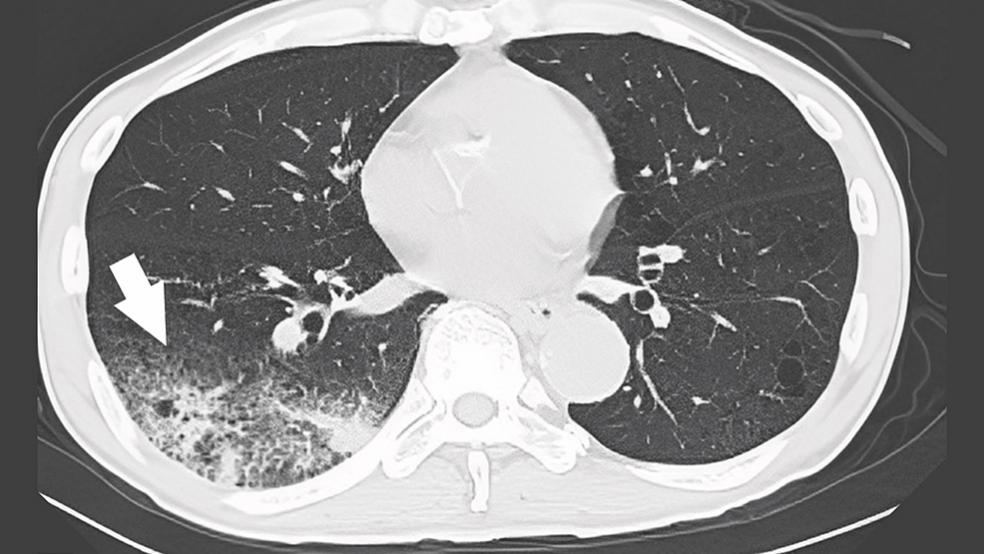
Results of chest computed tomography (day 1) showing right lower lobe infiltration
